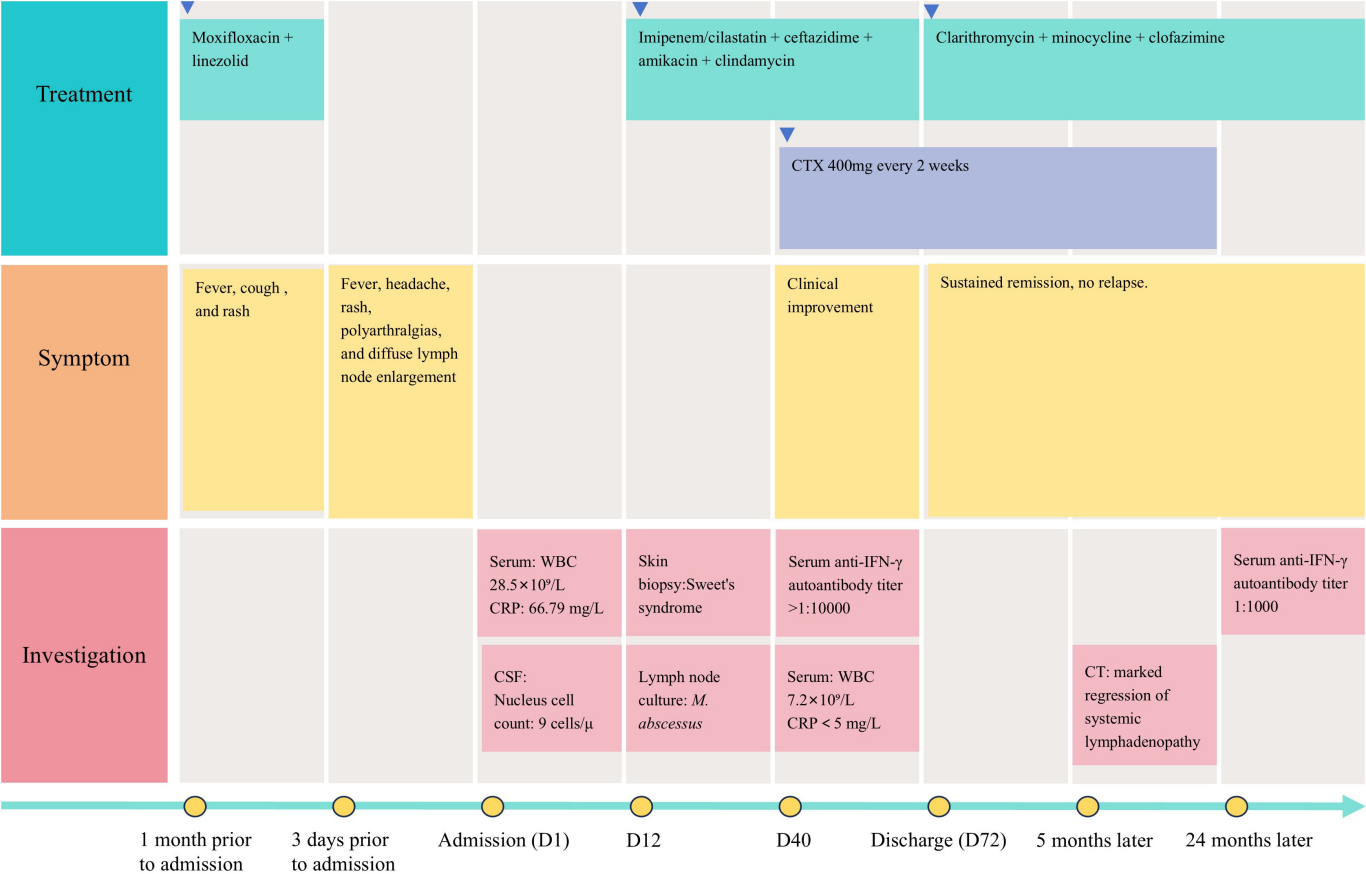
Timeline of the patient’s clinical trajectory and therapeutic Interventions. WBC, white blood cell count; CRP, C-reactive protein; CSF, cerebrospinal fluid; D, day; CTX, cyclophosphamide; CT, computed tomography.
